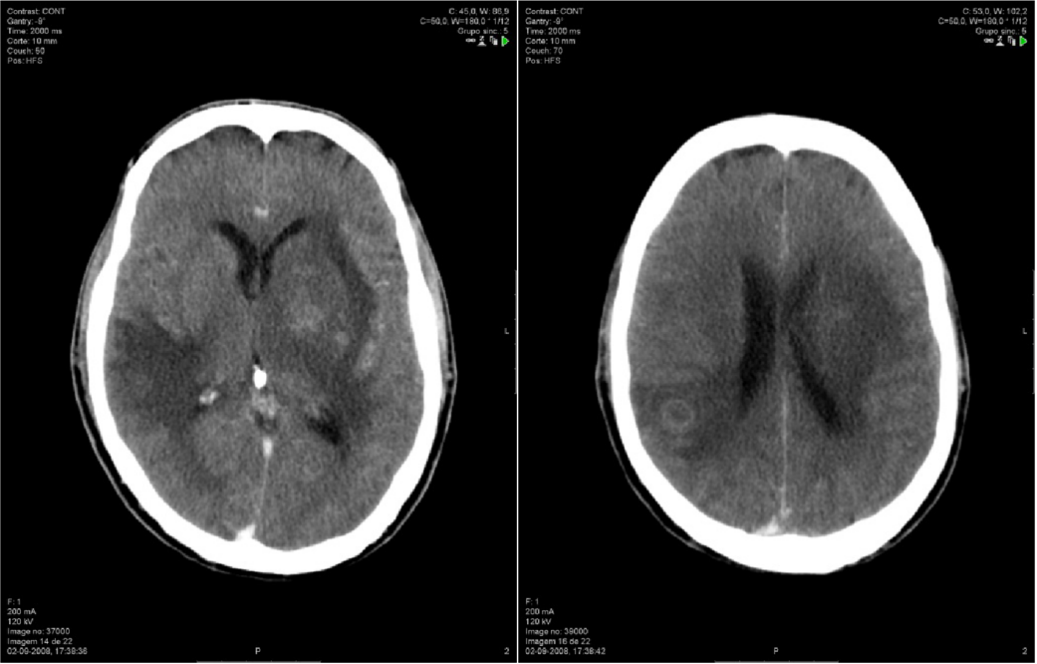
Head computed tomography
